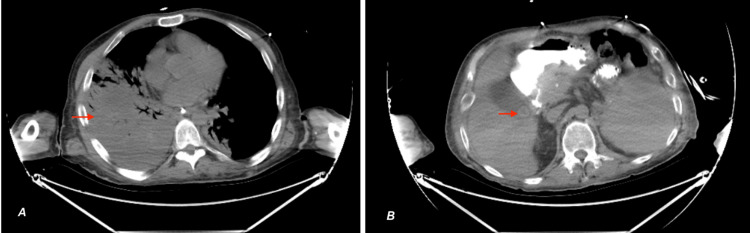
Axial CT image of the abdomen and pelvis without contrast demonstrating (A) multilobar consolidations and (B) stone in the gallbladder neck CT, computed tomography
